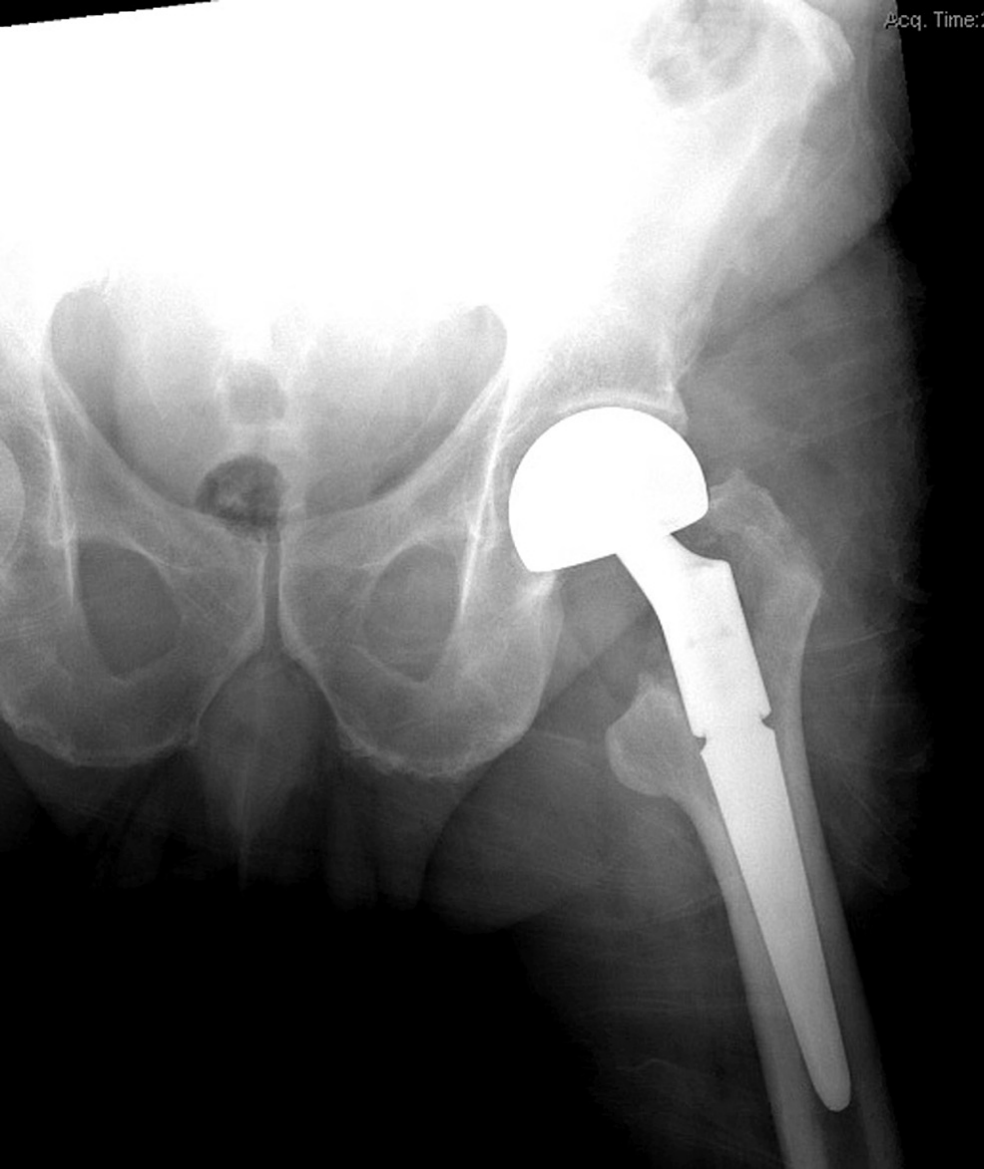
Press Fit hemiarthroplasty with a bipolar head. Initial postoperative X-ray of the left hip after distally fixating hemiarthroplasty.
